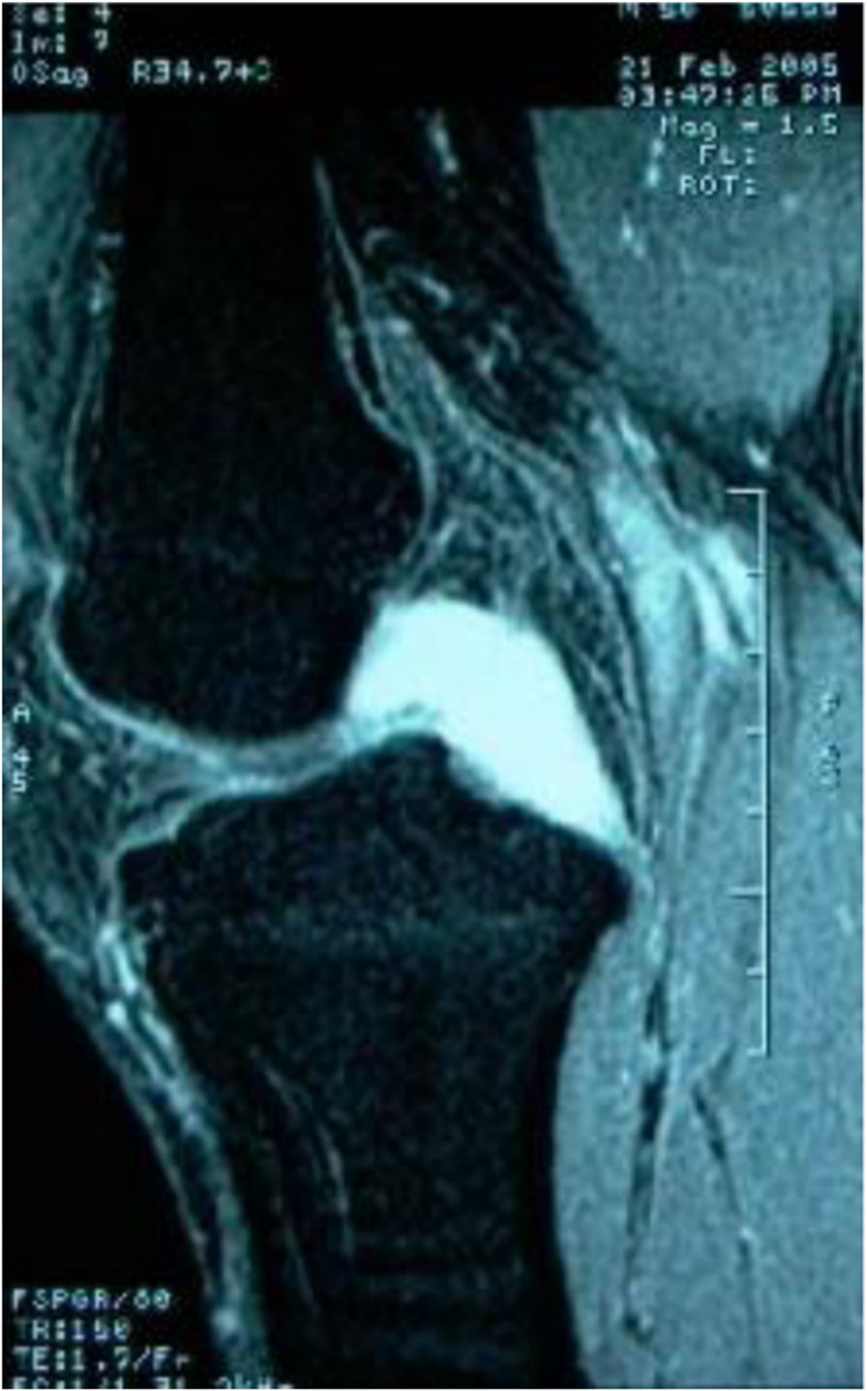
Sagittal fat-suppressed T2-weighted MR image showed hyper-intensity lesion with distinct border localized in posterior cruciate ligament.
